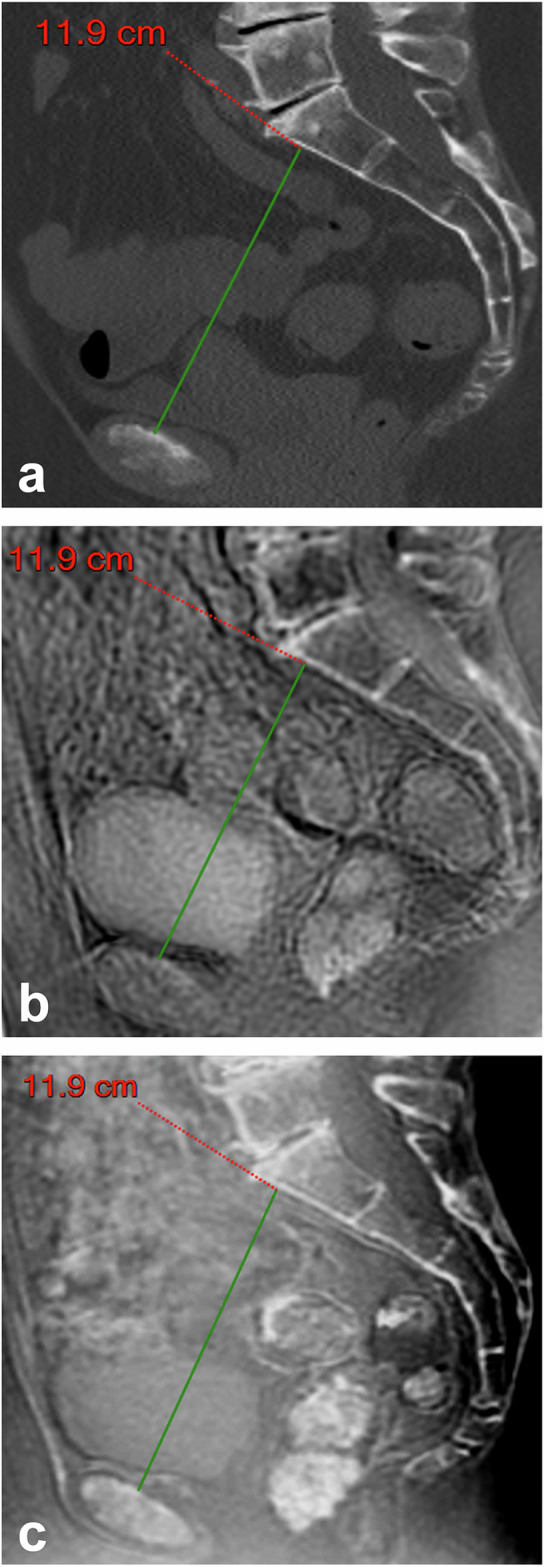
A 67-year-old woman with multiple myeloma, sagittal reformatted images for measuring the obstetric conjugate diameter (green line): (a) reference computed tomography; (b) inverted gray-scale zero echo time MRI sequence; and (c) inverted gray-scale black bone MRI sequence. MRI, Magnetic resonance imaging
